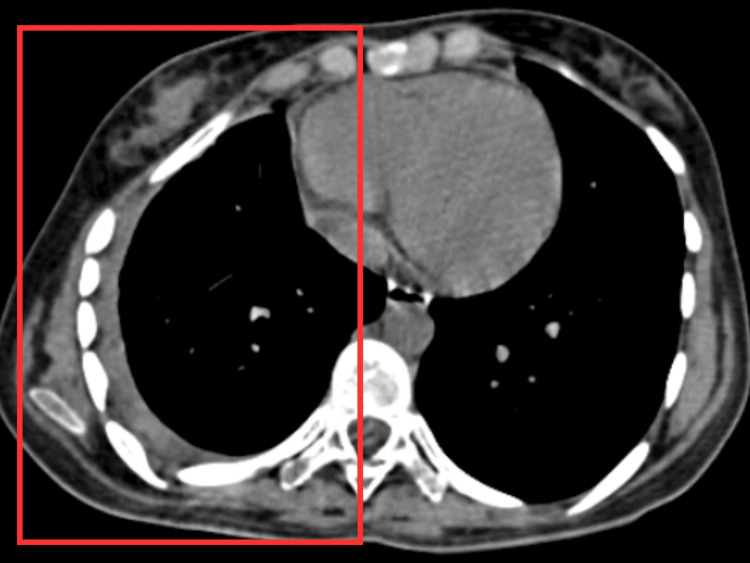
HRCT thorax HRCT: high-resolution computed tomography The square shows an ill-defined heterogeneously hyperdense lesion with hypodense areas and multiple calcific foci measuring approximately 7.4 × 5.8 × 5 cm [craniocaudal (CC) × transverse (TV) × anteroposterior (AP)] in right upper lobe adjacent to 1st and 2nd ribs, causing mild sclerosis of 1st rib
